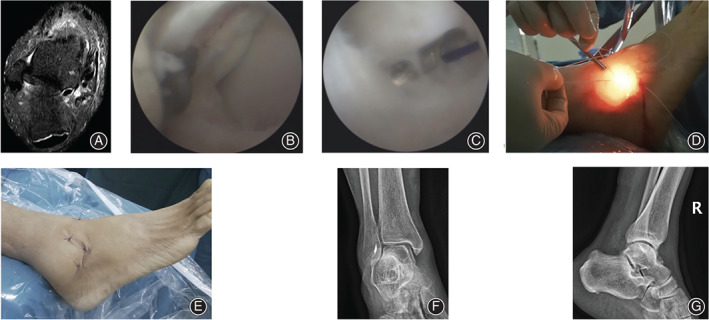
A 40‐year‐old female patient with chronic lateral ankle instability. (A) The preoperative MRI showed the integrity of the ATFL was interrupted of the right ankle. (B) The intra‐operative view under the arthroscope showed a suture anchor was introduced into the distal fibular. (C) A suture passer with PDS II was used to pass through the ATFL and inferior extensor retinaculum. (D) The anchor arms were sutured by free‐edge suture fashion. (E) The postoperative appearance of the portals. (F) The anterior–posterior X‐ray film of the right ankle after surgery. (G) The postoperative lateral X‐ray film of the involved ankle.
